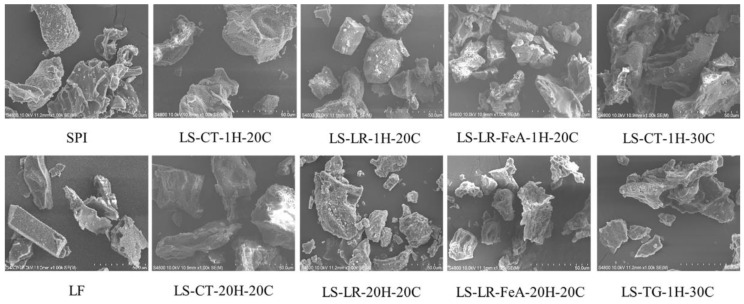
Morphology of lupin flour (LF), soy protein isolate (SPI), non-enzyme-treated LF and SPI mixture (LS-CT), and enzyme-treated mixtures with laccase (LS-LR) and transglutaminase (LS-TG) at 20 °C and 30 °C, respectively. FeA—Ferulic acid-added; H—Treatment time (h); C—Incubation temperature (°C).
